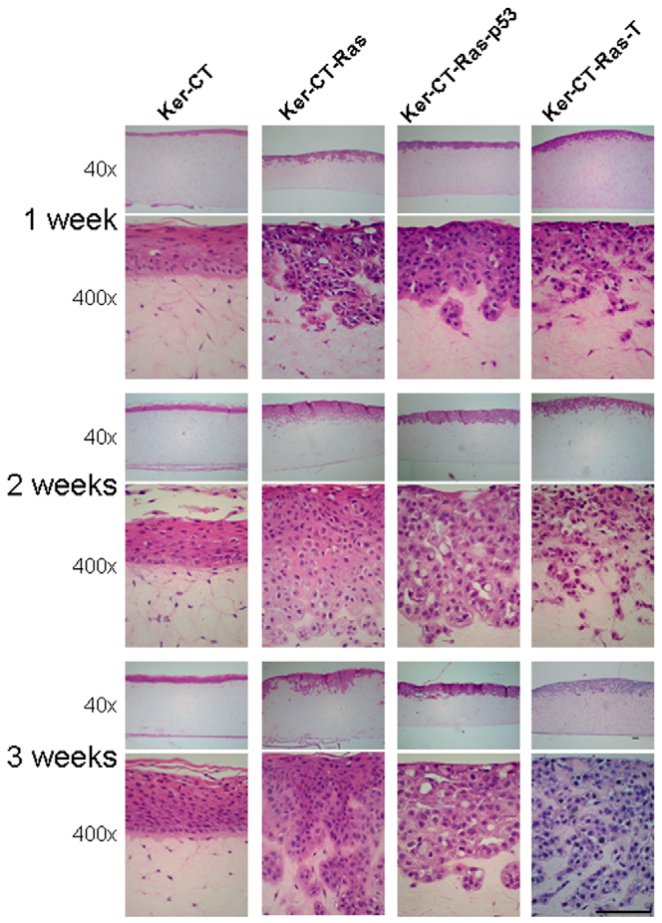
Effect of time on epithelialization. All skin equivalents demonstrated a stratified epithelium on the upper surface within one week. With H-Ras overexpression keratinocyte invasiveness typically occurred early and slowed with time. Similar results occurred with all H-Ras permutations. All but Ker-CT-Ras-T retained some ability to cornify. Upper images, 40× total magnification; lower images, 400× total magnification. Scale bar: 100 µm.
